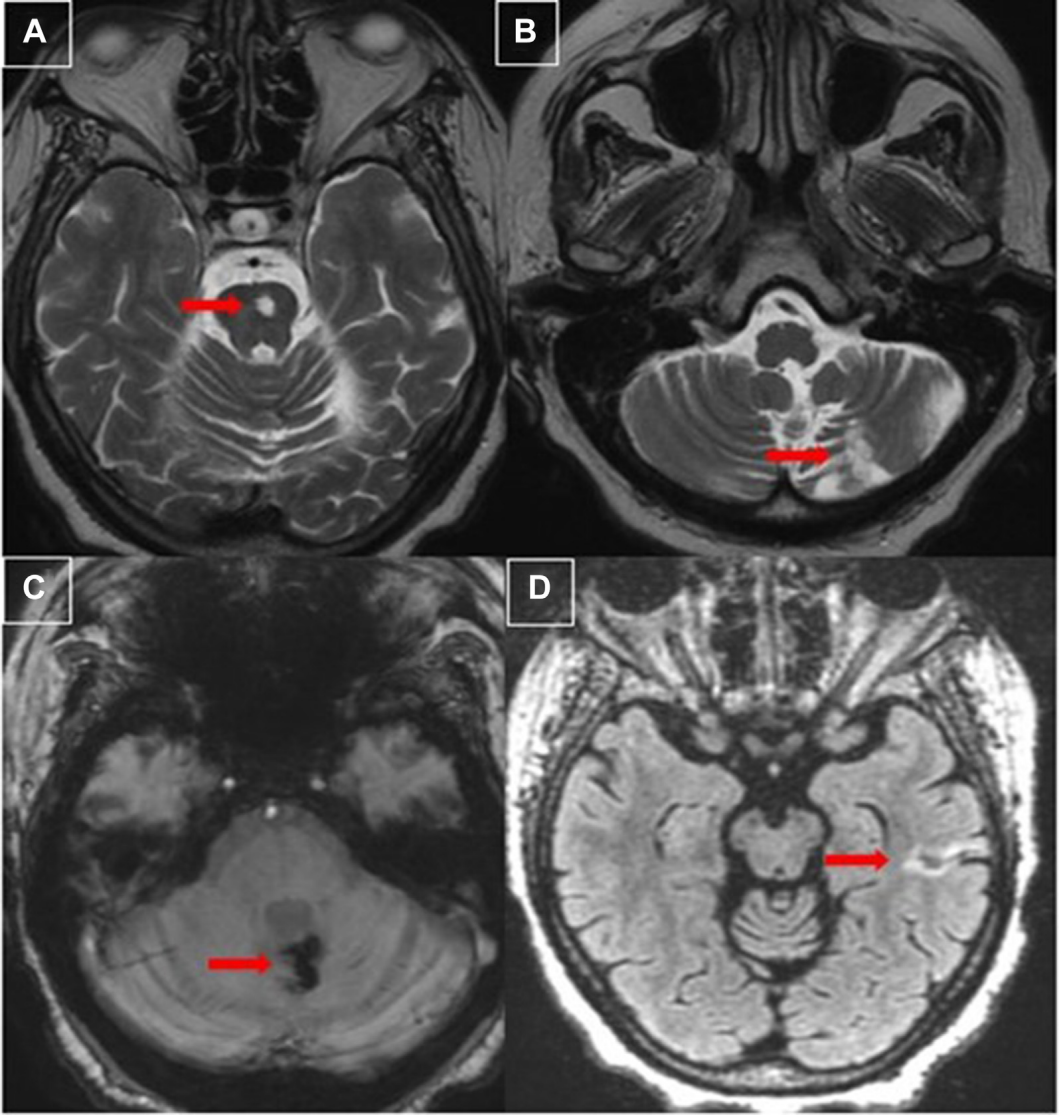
Head MRI (A) Axial T2 weighted sequencing, chronic left pontine infarction with cavitation (red arrow). (B) Axial T2 weighted sequencing suggests chronic infarction of the left cerebellar hemisphere consistent with prior embolism (red arrow). (C) Susceptibility weighted imaging axial reconstruction demonstrates foci of hemosiderin deposition in the left cerebellar hemisphere and vermis consistent with hemorrhagic transformation of a prior ischemic infarct (red arrow). (D) Axial T2 weighted Fluid Attenuated Inversion Recovery (T2 FLAIR) demonstrates gliosis of the left inferior temporal lobe consistent with chronic infarction (red arrow). MRI = magnetic resonance imaging.
